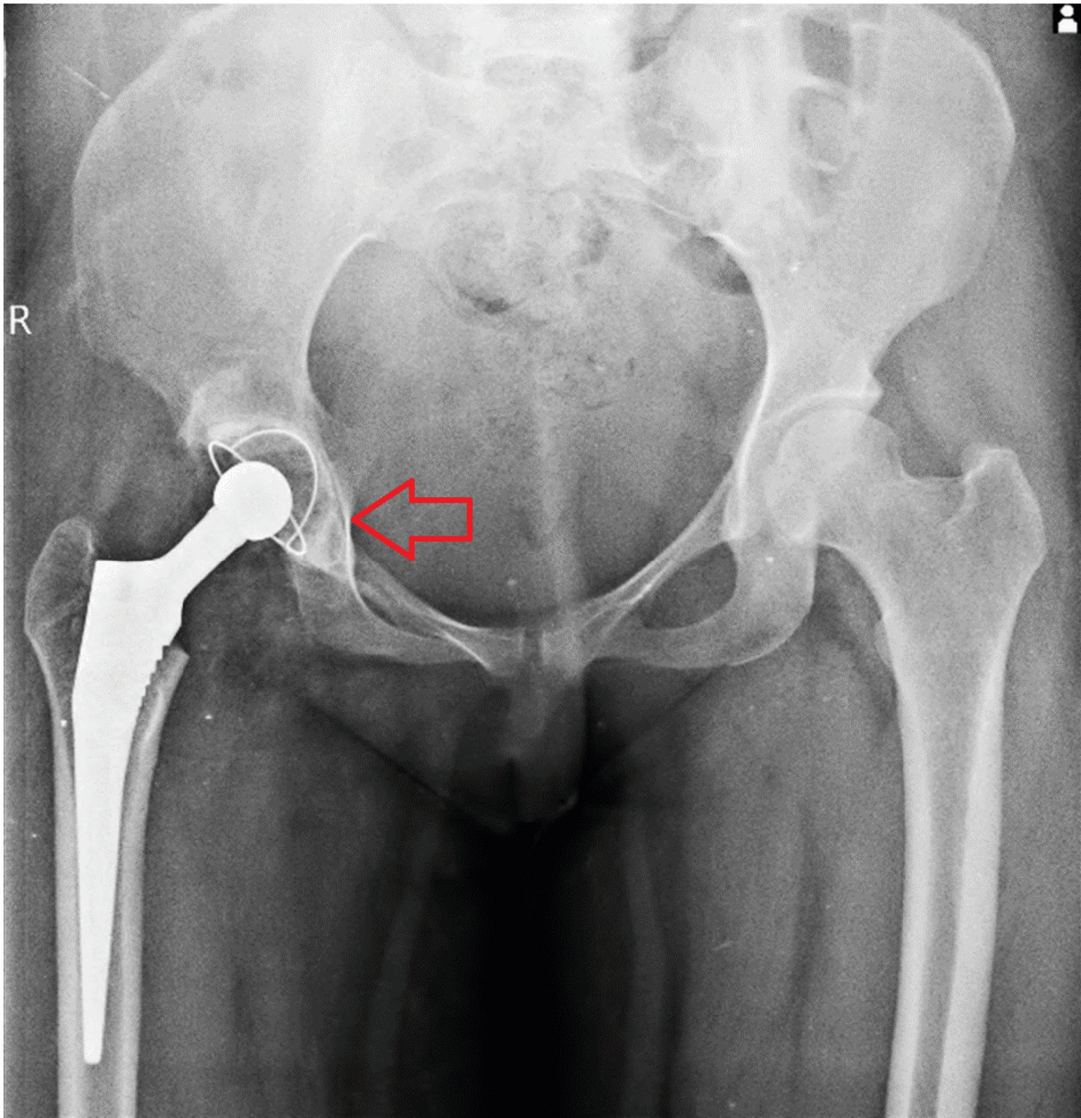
Postoperative follow-up radiograph of the pelvis with both hips' anteroposterior view showing stable total hip arthroplasty prosthesis in situ.
